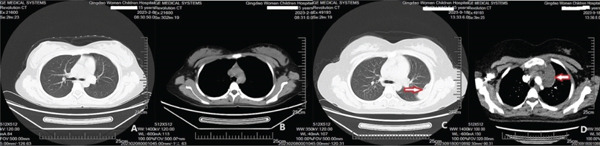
Chest CT in September revealed new pleural and mediastinal masses (C‐D) that were not present on imaging seven months prior (A‐B).
